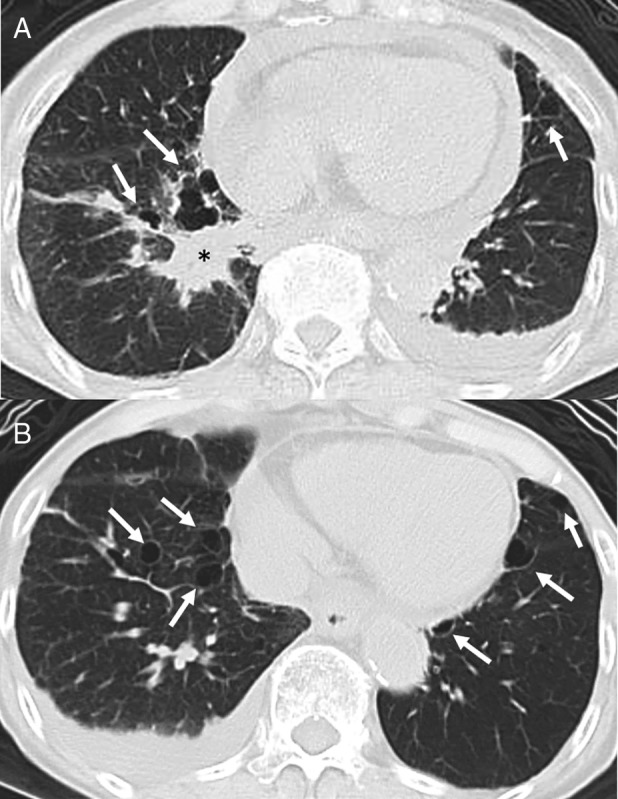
Chest computed tomography (CT) of the 89‐year‐old female patient (mother). Bilateral multiple lung cysts (arrows) were located in the basilar medial regions of the lung. A mass (*) was observed in the right lower lobe, with bilateral pleural effusion and pericardial effusion.
